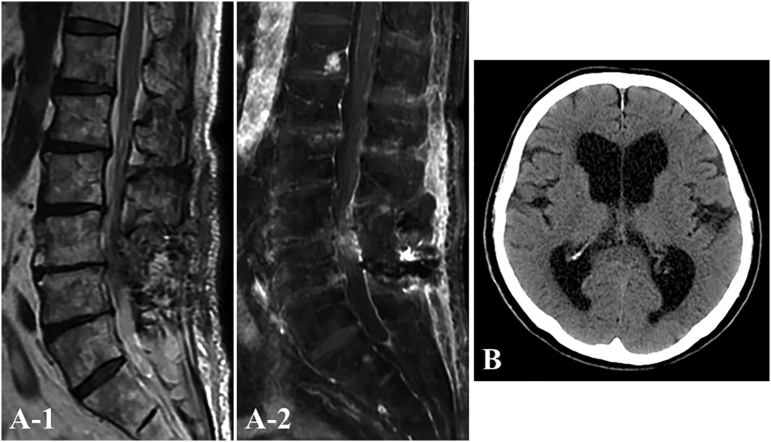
Postoperative sagittal T2-weighted (A-1) and Gd-enhanced T1-weighted (A-2) MRI after surgical resection show no residual tumor. CT after 4 months (B) demonstrates a slight reduction in ventricular size and clear depiction of the cerebral sulcus.
